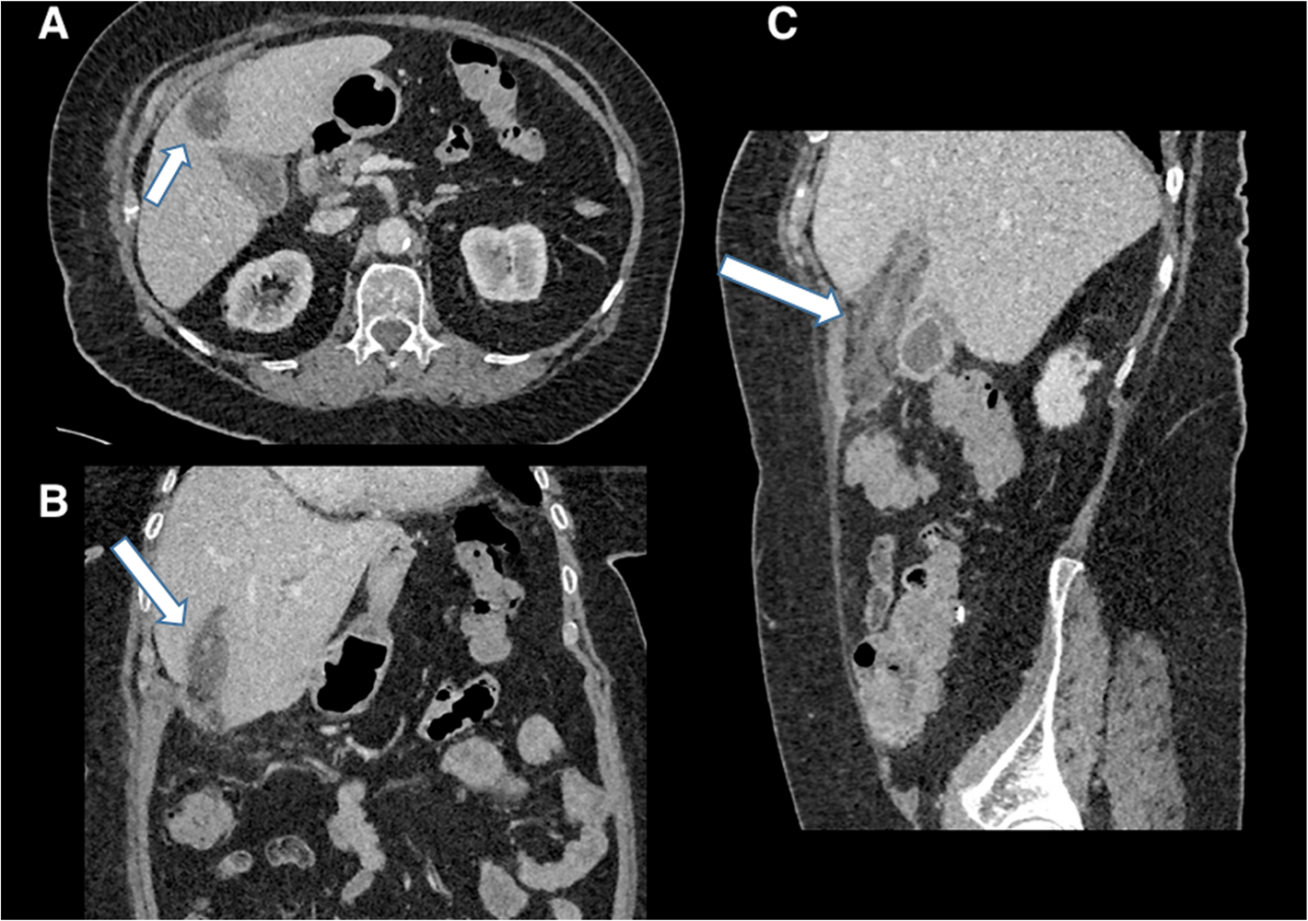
Axial (a), coronal (b) and sagittal (c) contrast-enhanced CT of the abdomen. There is gallbladder wall thickening and pericholecystic fluid consistent with acute cholecystitis. There is marked expansion and oedema surrounding the falciform ligament (arrows). Appearances are due to thrombophlebitis of a recanalised umbilical vein
